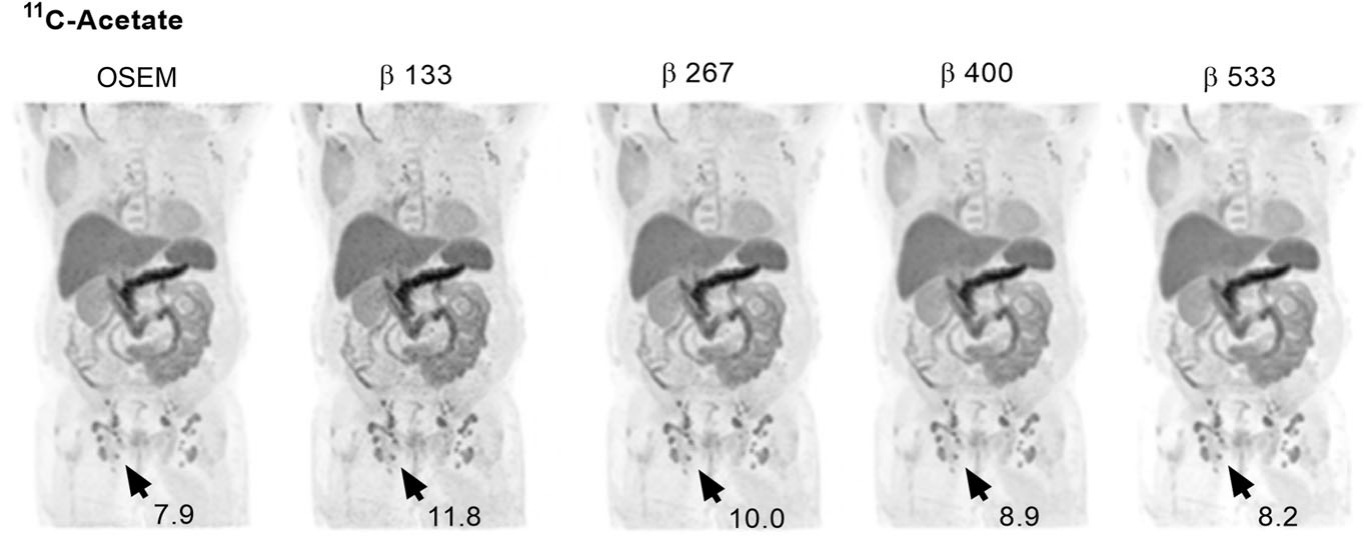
Coronal whole-body ^11^C-acetate PET images of a patient with soft tissue prostate cancer metastases. Images were acquired 11 min post-injection and reconstructed with 3 min/bed using OSEM (3 iterations, 16 subsets, 5-mm Gaussian post-processing filter) and BSREM with *β*-values of 133, 267, 400, and 533. Both reconstruction methods included TOF and PSF recovery. The administered activity was 4.2 MBqkg^−*1*^. The SUV_max_ of the lesion (arrow) is shown for the respective reconstruction.
